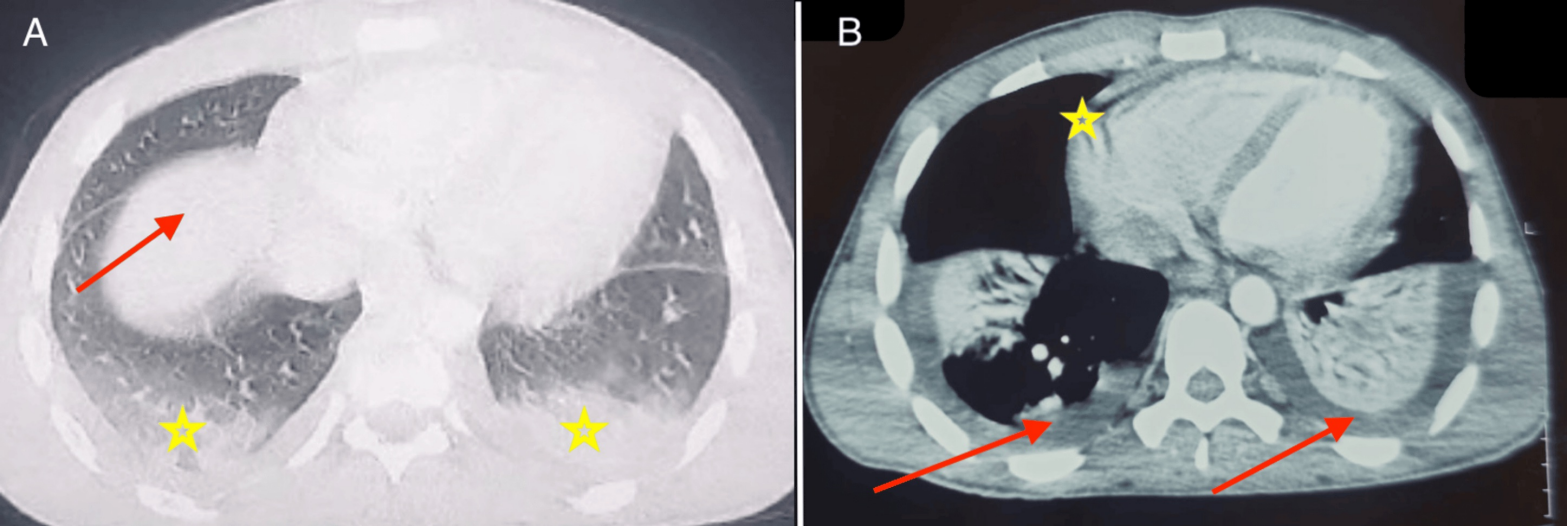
Axial view of contrast-enhanced thoracic CT scan A: Parenchymal window shows ground-glass hyperdensity in the right upper lobe (red arrow) with bilateral lower lobe pulmonary consolidation (yellow stars); B: Mediastinal window shows moderate bilateral pleural effusion (red arrows) with pericardial effusion (yellow star)
